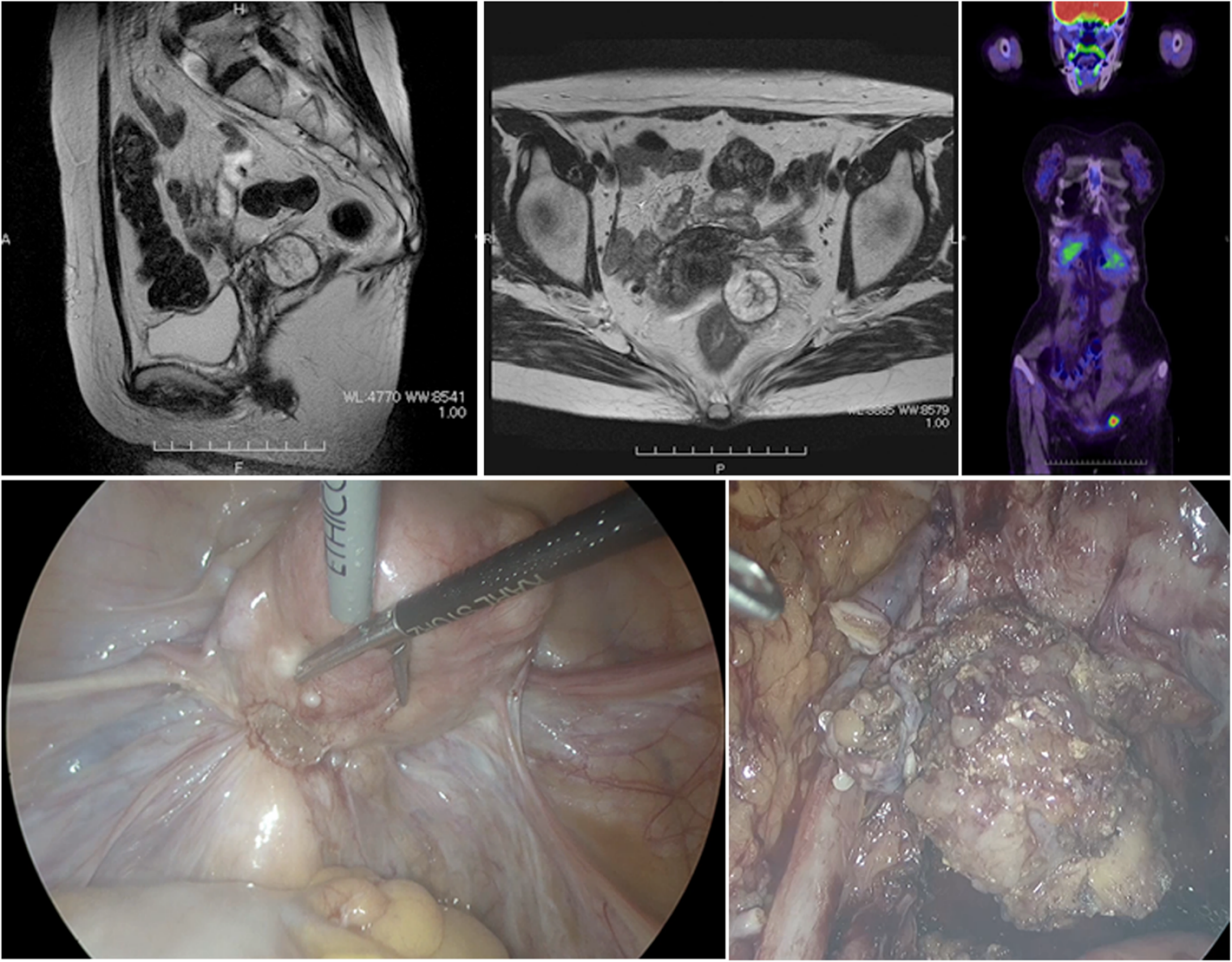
Magnetic resonance images and laparoscopic findings on the tumor located in the cul-de-sac. a Sagittal T2-weighted image. b Transverse T2-weighted image. c Whole-body ^18^F-fluorodeoxyglucose (FDG) positron emission tomography (PET) scan image. FDG-PET scan shows high FDG uptake at the left side of the pelvic cavity. d Photograph of laparoscopic findings of the cul-de-sac tumor in the left uterosacral ligament. e Photograph of the tumor excavated from the cul-de-sac
